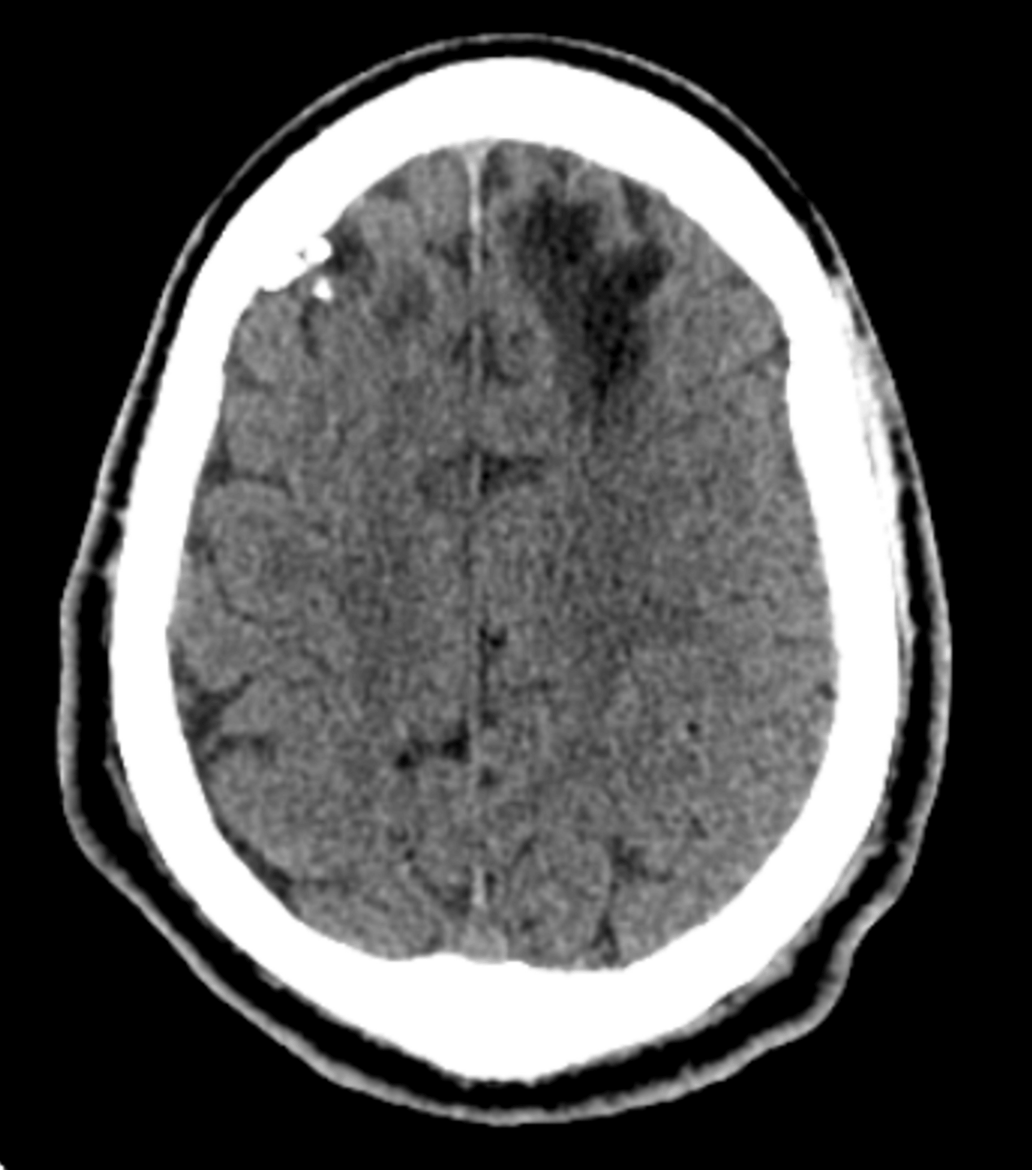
CT of the head without contrast showing the chronic hypodense focus of encephalomalacia in the left frontal lobe. Normal size and configuration of ventricles and cisterns; no mass effect or midline shift.
